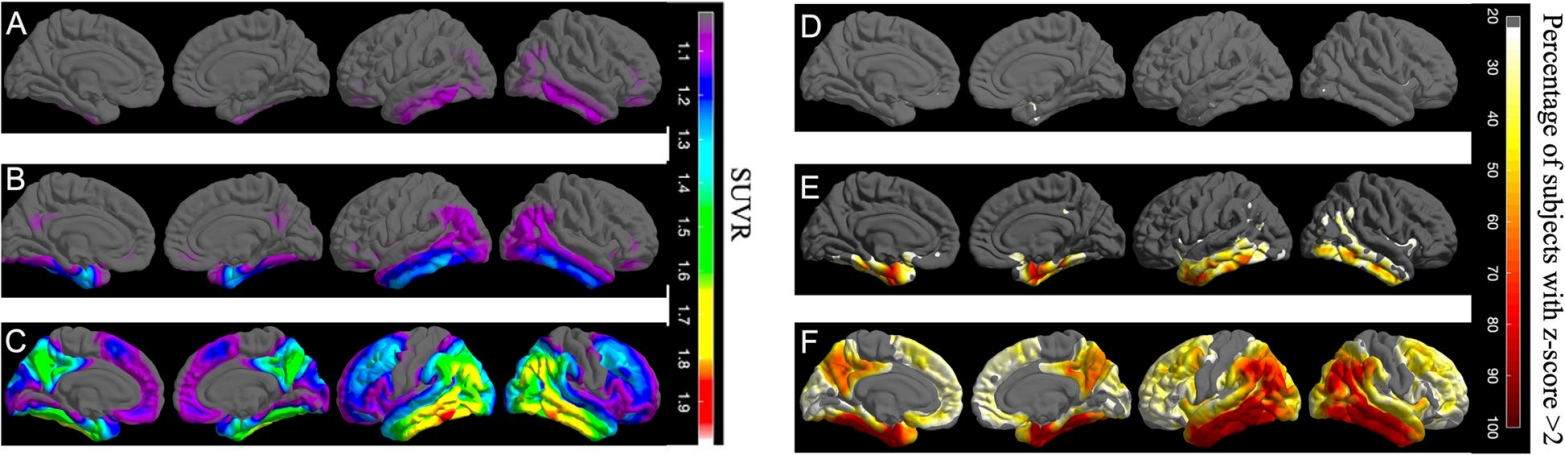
A The average SUVR surface map of Q3 (*Eτ*−/AD_signature_−) subjects, showing a lack of elevated flortaucipir signal in the entire cortex. B The average SUVR surface map of Q2 (*Eτ+*/AD_signature_–) subjects. There is an elevated flortaucipir signal primarily in the regions of *Eτ* VOI region with the exception of the left middle temporal gyrus. C The average SUVR surface map of Q1 (*Eτ+*/AD_signature_+) subjects. Widespread elevation of flortaucipir signal across the entire cortex with the exception of postcentral, precentral, and paracentral gyri and anterior cingulate. D–F Surface maps showing the percentages of subjects that are tau positive for all FreeSurfer regions in Q3 (*Eτ*−/AD_signature_−), Q2 (*Eτ+*/AD_signature_–), and Q1 (*Eτ+*/AD_signature_+), respectively
